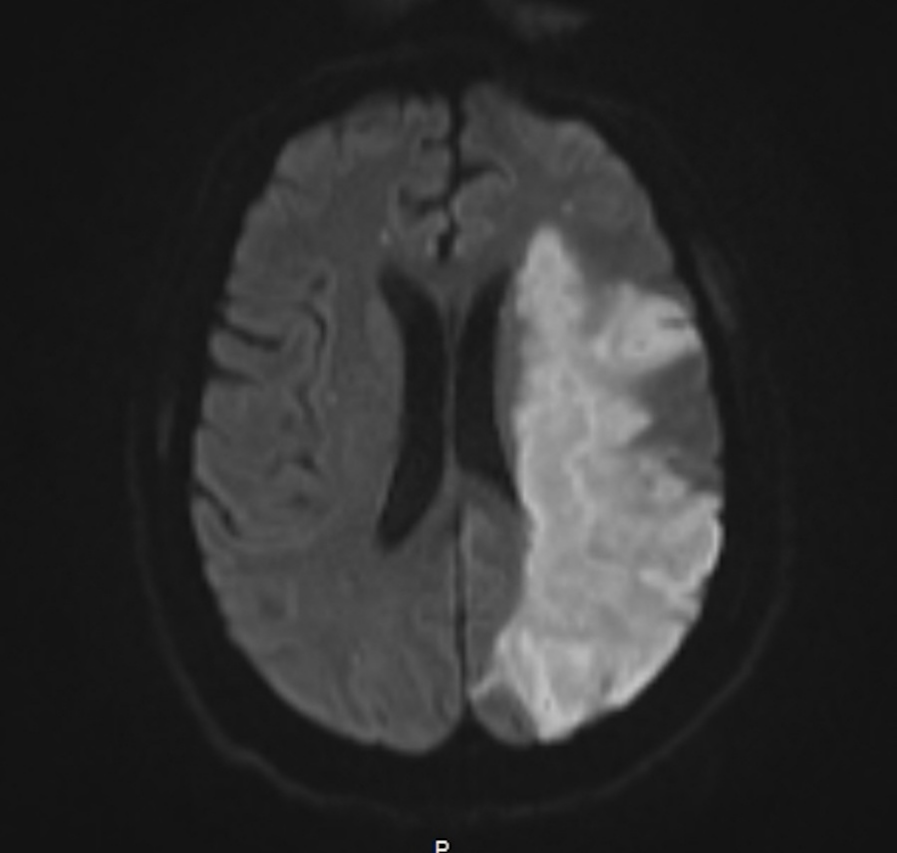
Magnetic resonance imaging (MRI) brain. Delayed MRI demonstrating large left middle cerebral artery (MCA) territory infarction.
